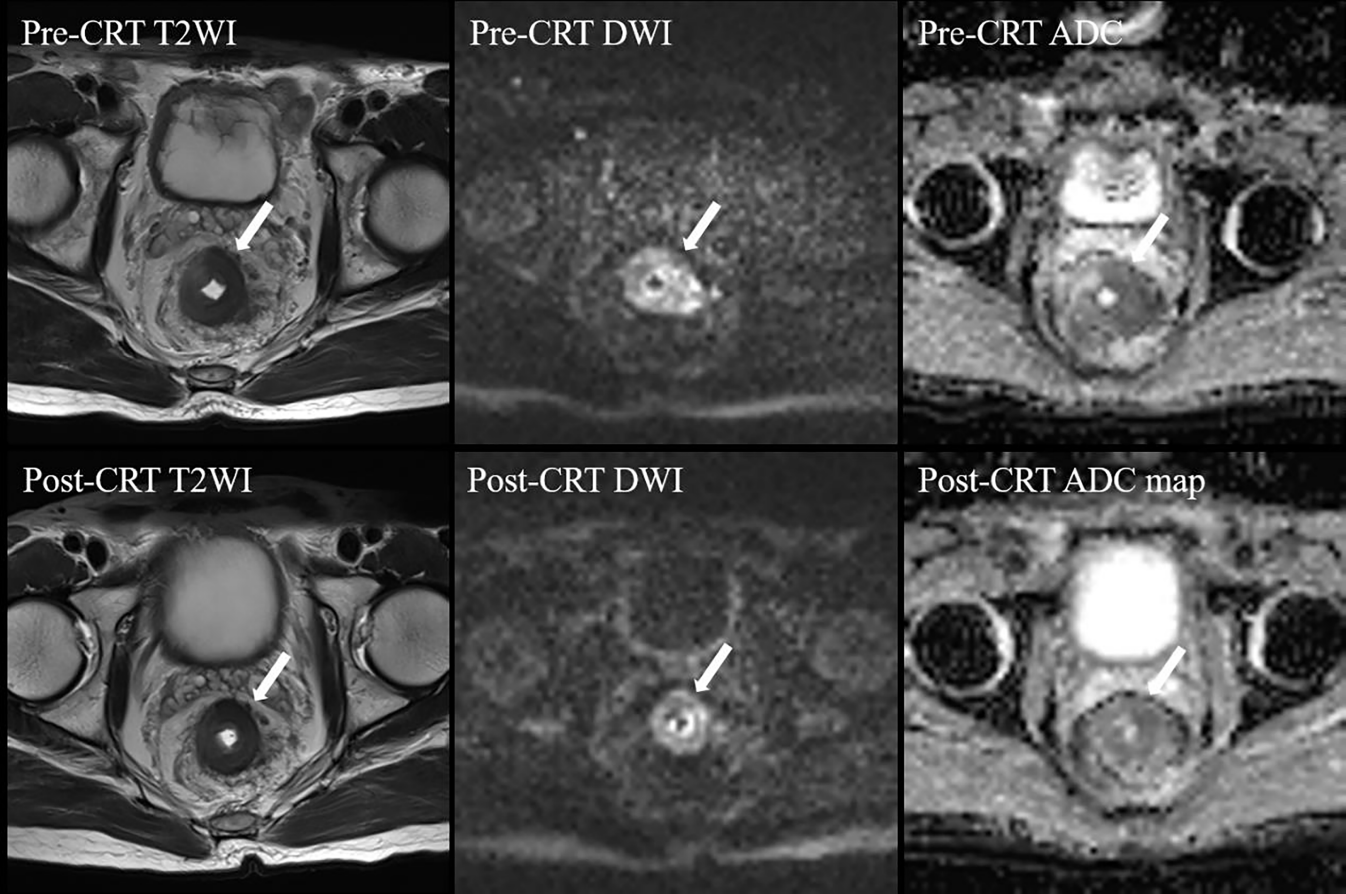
MRI tumor regression grade 4 in a 59-year-old man after CRT. Post-CRT T2WI shows a slight reduction (< 50%) in the tumor size with a majority of intermediate signal intensity. On high b value post-CRT-DWI, a thick layer of diffusion restriction was apparent at the tumor bed. CRT, chemoradiotherapy; T2WI, T2-weighed imaging; DWI, diffusion-weighted imaging; ADC, apparent diffusion coefficient.
